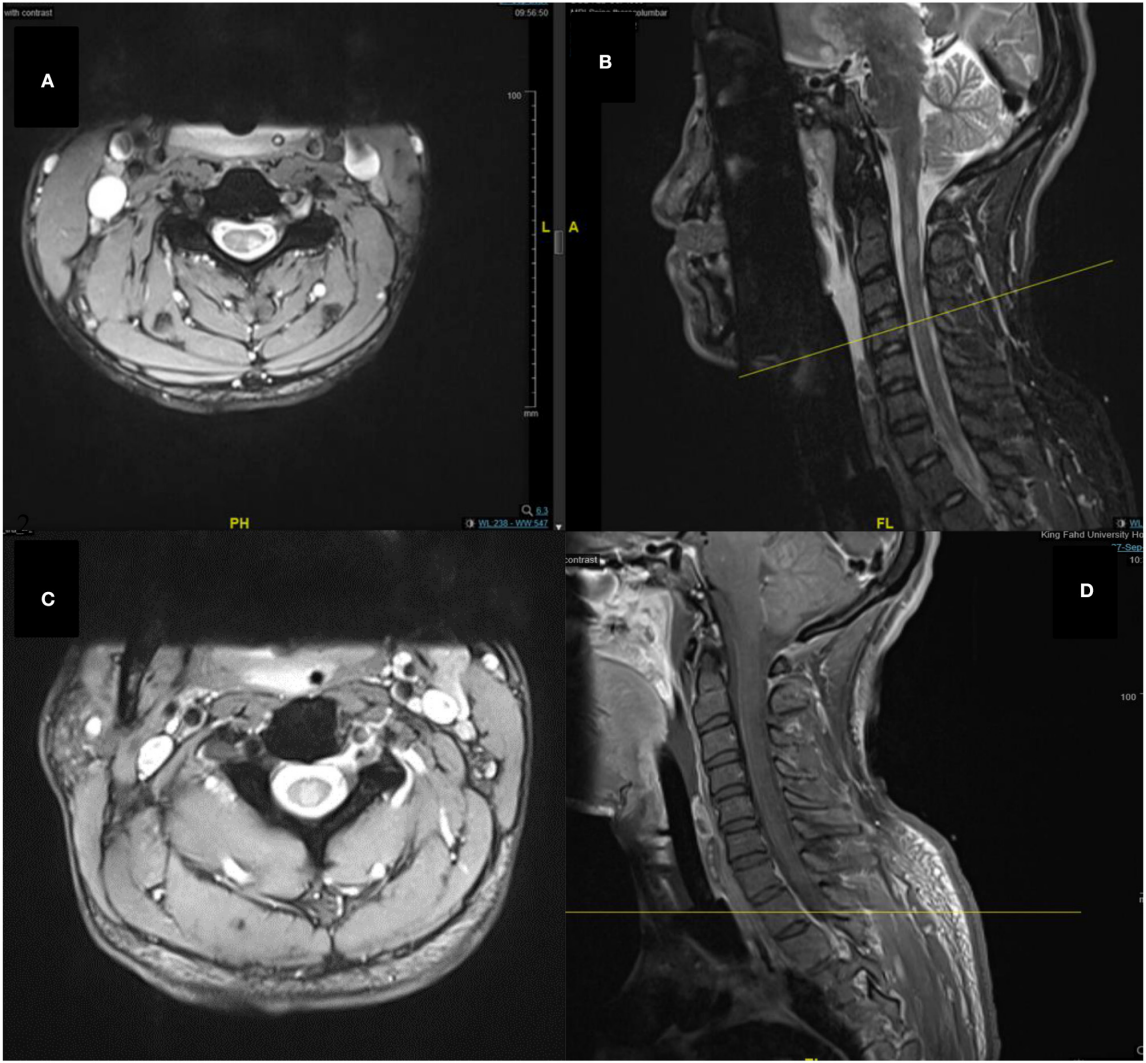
Spine MRI images of T2-weighted (A), sagittal short tau inversion recovery (STIR) (B), T2-weighted (C), and sagittal T1-weighted with contrast (D) sequences. Showing multiple abnormal high signal intramedullary lesions at the central, posterior, and lateral aspects of the cervical and thoracic spinal cord.
